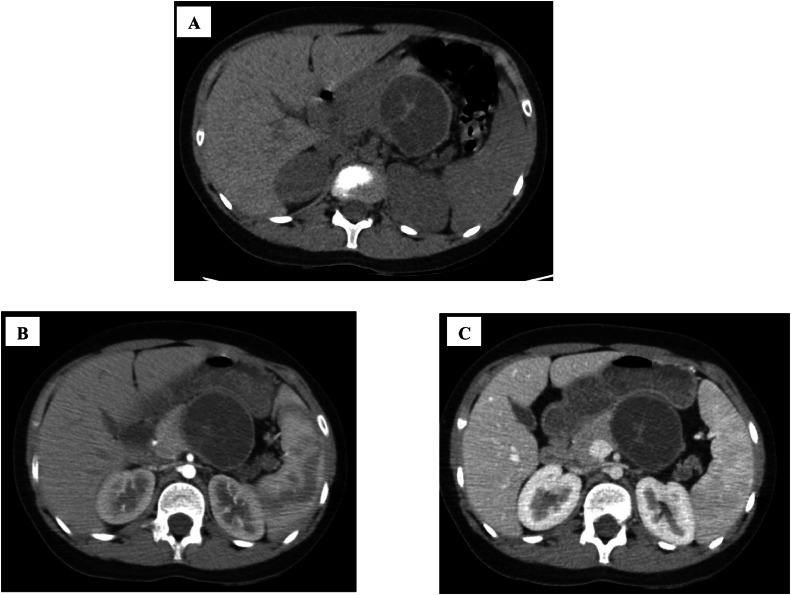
(A) Axial CT scan without contrast, (B) Axial contrast enhanced CT scan on arterial phase, and (C) on portal venous phase reveals a multi loculated fluid attenuated lesion (with average attenuation of 8 HU) in pancreatic body containing non enhancing relatively hyperdense septa.
